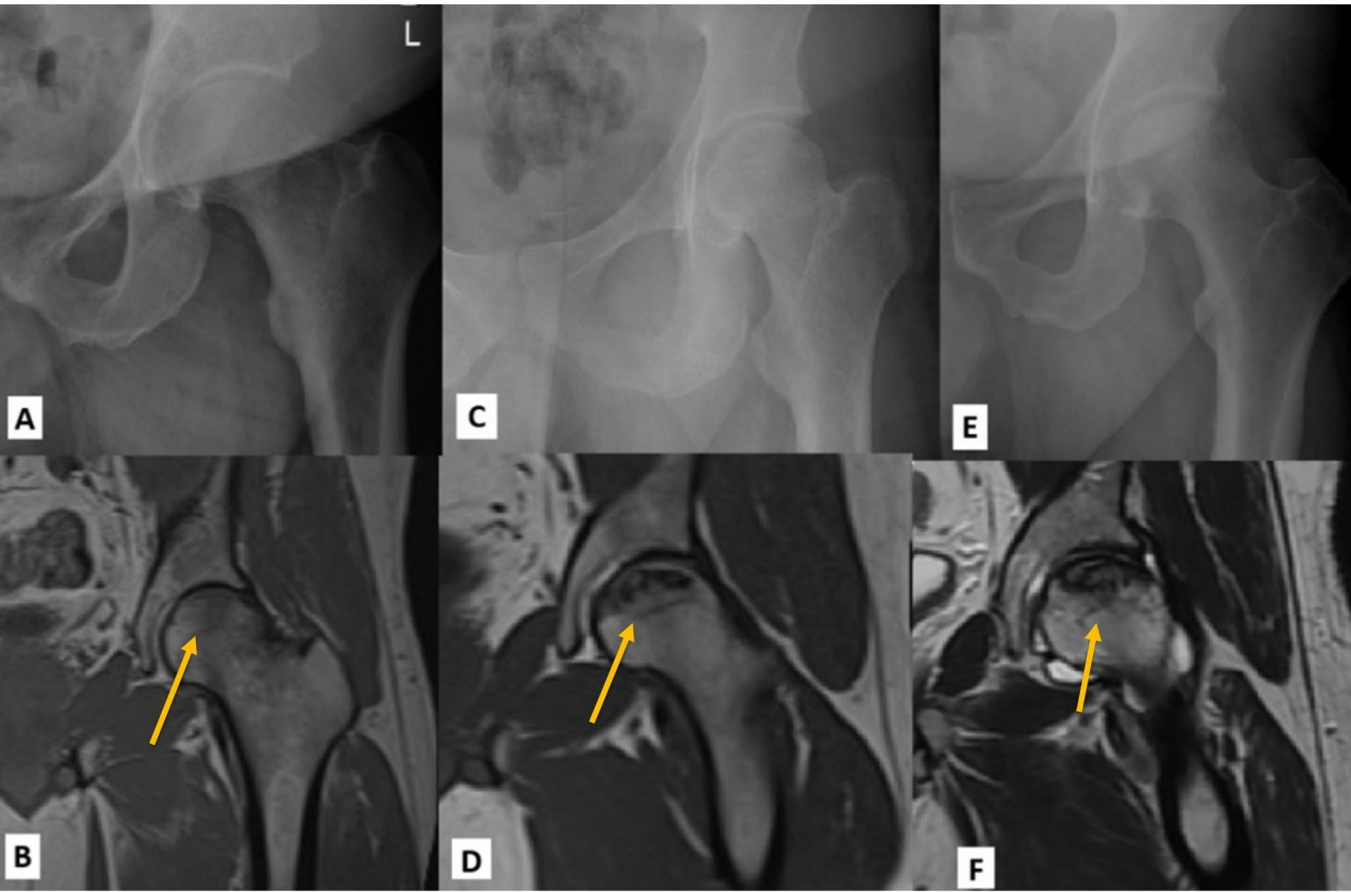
Radiographic progression of AVN of the hip through Ficat stages 1, 2, and 3. (A) AP of the left hip of a patient diagnosed with Ficat stage 1 AVN. The plain radiograph appears normal. (B) Coronal T2 fat-suppressed MRI of the left hip in the same patient with evidence of Ficat stage 1 AVN of zone 3 of the femoral head characterized by focal marrow edema. (C) AP of the left hip of a patient diagnosed with Ficat stage 2a AVN. The plain radiograph shows mild subchondral cysts without any subchondral lucency or cortical collapse. (D) Coronal T1 weighted MRI of the left hip in the same patient with evidence of Ficat stage 2a AVN characterized by geographic signal changes in zones 2, 3, and 4 and a sclerotic reactive margin. There is no evidence of cortical collapse. (E) AP of the left hip of a patient diagnosed with Ficat stage 2b AVN. The plain radiograph shows a subtle crescent sign. (F) Coronal T2 weighted MRI of the left hip in the same patient with evidence of Ficat stage 2b AVN characterized by geographic signal changes in zones 2, 3, and 4 and mild cortical collapse with intact overlying cartilage
